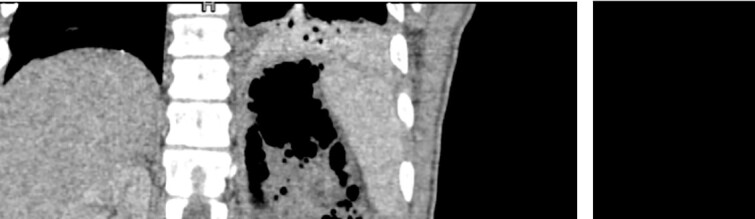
CT images of the abdomen (venous phase) revealed a protruberance in the left inguinofemoral region extending into the retroperitoneal space, progressing as a perirenal abcess, spreading to the left sub-diaphragmatic region and causing an air-fluid level and an infiltrate in the lower lobe of the lung.
